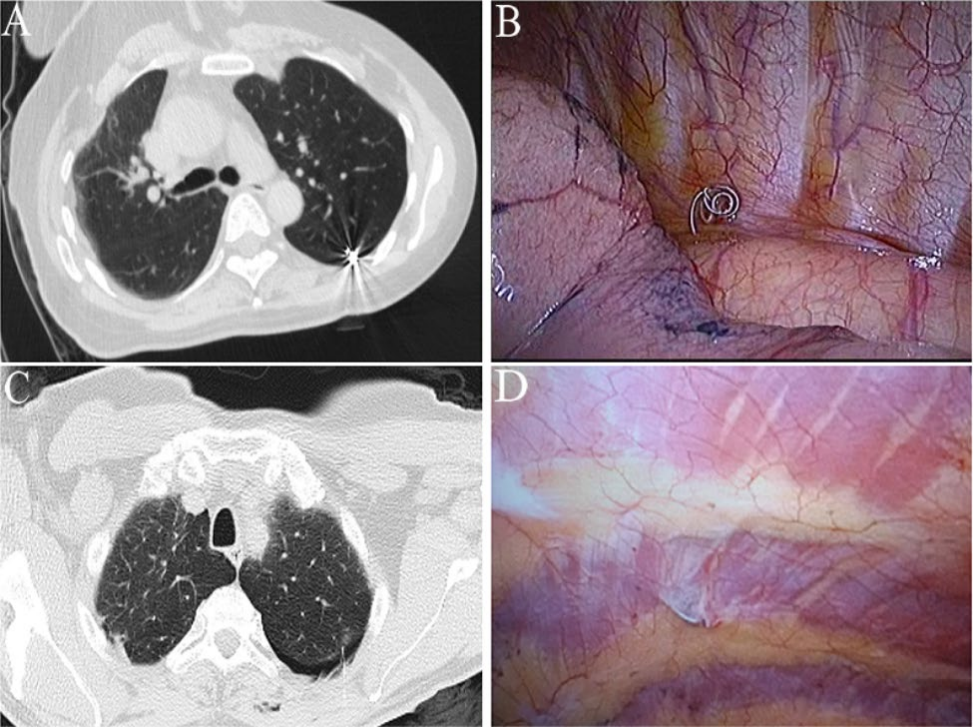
Images of lung CT scan and surgical field for placement and fixation of marking materials. (A) The coil was placed into a GGN adjacent to the pleura. (B) The coil was fixed to the chest wall. (C) The tip of the hookwire was placed into a GGN at the left lung. (D) The hookwire was fixed to the chest wall
